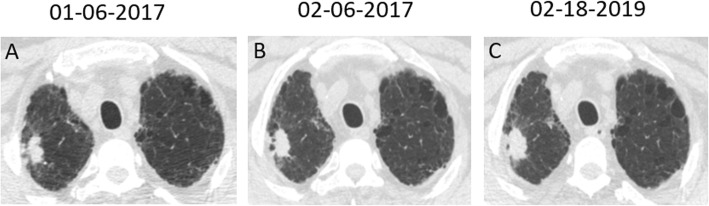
Serial chest CT scan showing enlargement of nodular opacities in the right upper lobe. *Mycobacterium tuberculosis* was cultured from the sputum sample submitted at the last evaluation. He was diagnosed as having pulmonary TB
